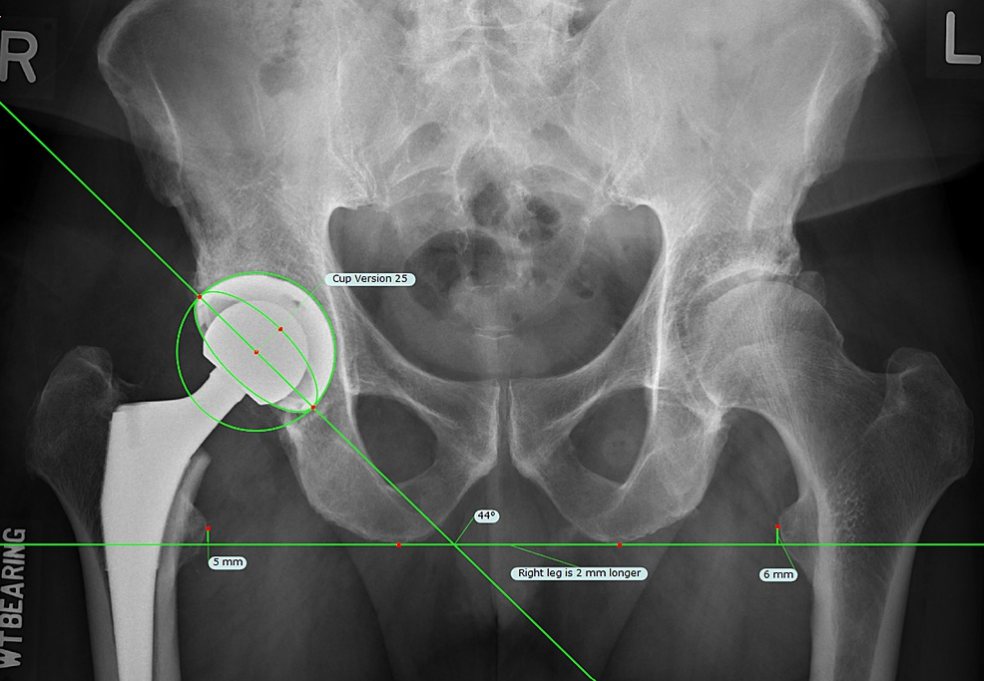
Primary reference value. The primary reference value was obtained from radiographic measurement using TraumaCad.
